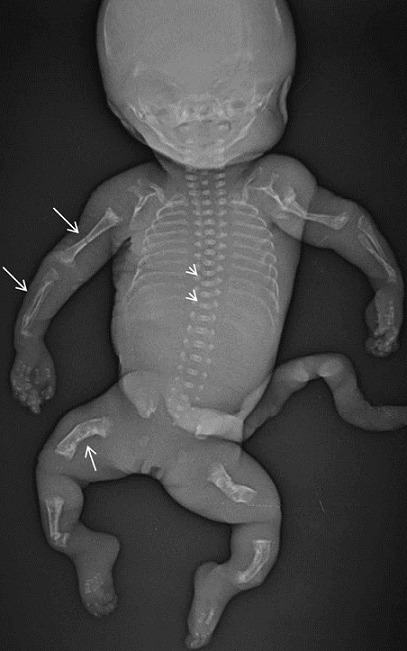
Radiographie du squelette fœtal: fractures multiples des os longs avec déformation des fémurs en receveur téléphonique en rapport aux cals osseux (flèche blanche). Corps vertébraux aplatis (tête de flèche)
